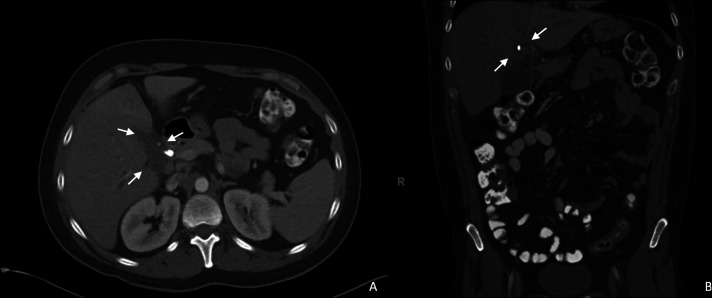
A CT scan of the abdomen and pelvis before endoscopic retrograde cholangiopancreatography. (A) Axial image demonstrating 5.3 by 3.5 by 3.2 cm ill-defined hypoattenuating lesion at the porta hepatis. (B) Coronal image demonstrating intrahepatic biliary ductal dilation proximal to the lesion.
